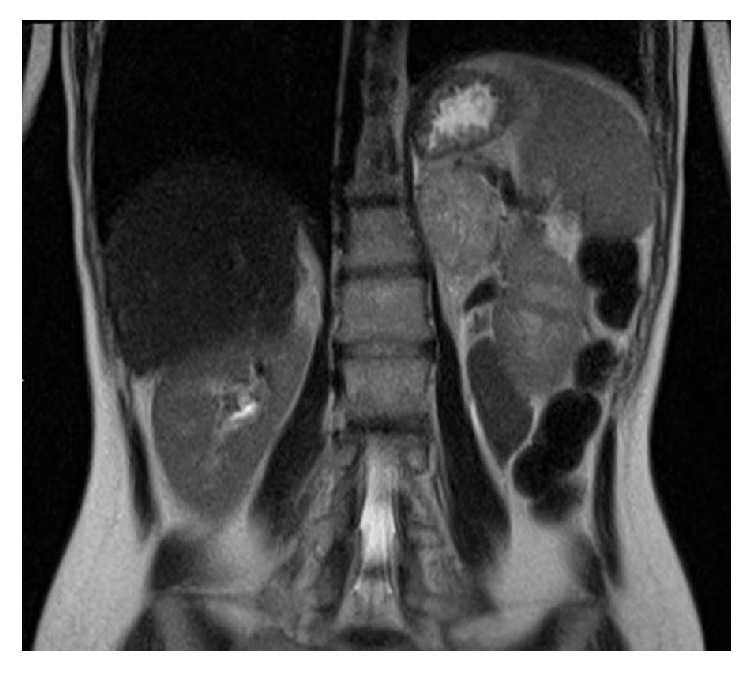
Abdominal MRI scan: presence of a big heterogeneous adrenal lesion, with hyperintense spots due to hematic content.
